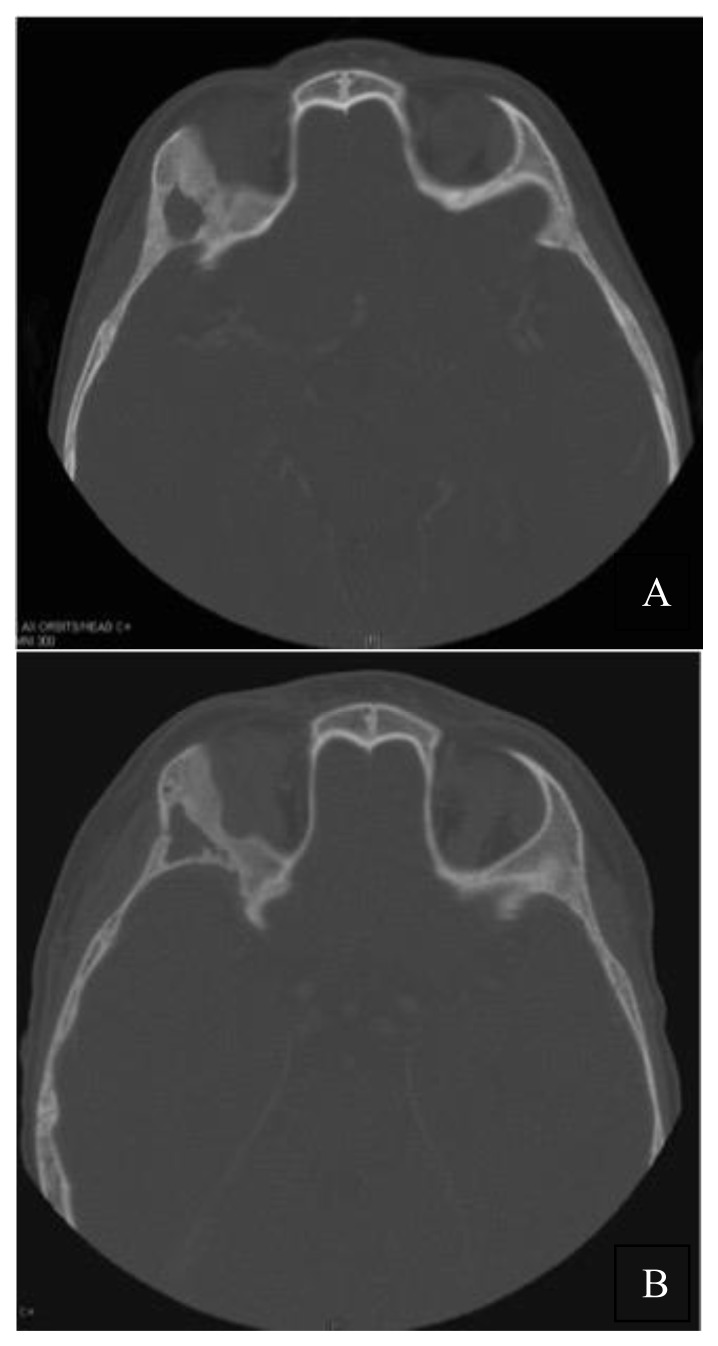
Skull CT before (a) and after (b) 2 courses of pamidronate therapy. a) Permeative bone lesions in the lateral wall of orbit and soft tissue mass involving the right lacrimal gland and lateral rectus muscle. b) Bone remodeling and reduction in orbital soft tissue mass
